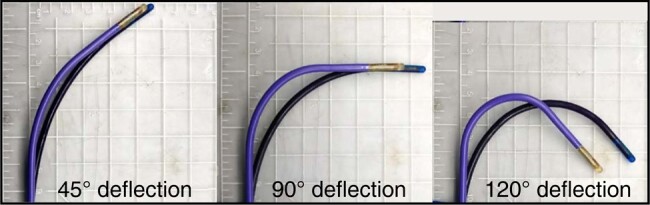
Deflection comparison between ACUNAV™ (black, Biosense Webster, Irvine, CA) and NUVISION™ (purple, Biosense Webster, Irvine, CA) ultrasound catheters. Deflections were conducted at room temperature. Modified and reproduced with permission from Biosense Webster, Irvine, CA.
